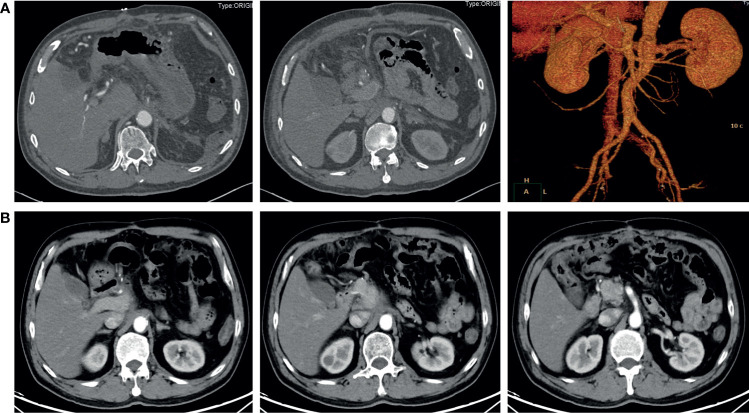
Contrast-enhanced abdominal CT after surgery. (A) 6 days after the radical surgery. The distal pancreas, spleen, and celiac trunk were absent. (B) 8 months after the radical surgery. No signs of recurrence or metastasis were found.
